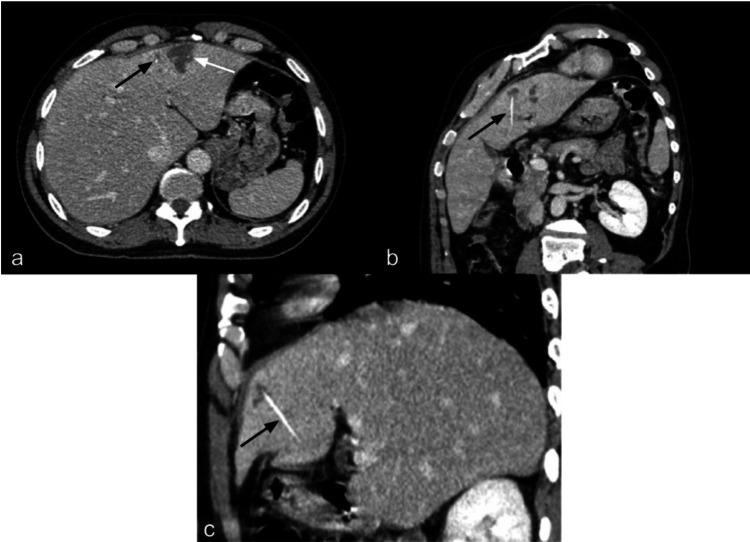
Second abdominal contrast-enhanced computed tomography scan showing a relapse of the left lobe abscess. (a) Transversal view showing a left lobe abscess (white arrow) and a radiodense structure (black arrow); (b) Coronal view showing a radiodense linear structure (black arrow); (c) Sagittal view showing a radiodense linear structure (black arrow).
